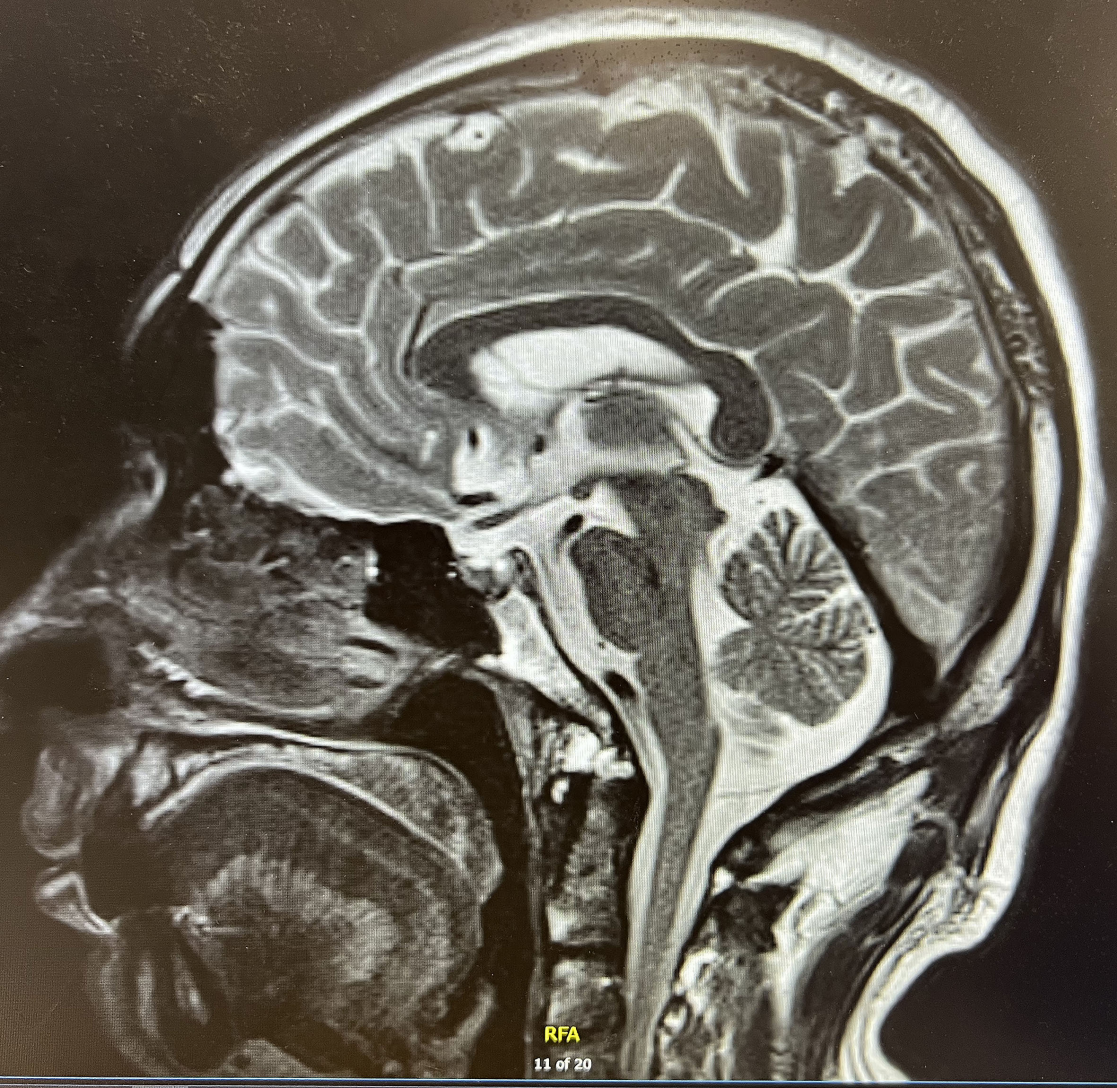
Post-contrast T2-weighted MR image of the pituitary gland.
